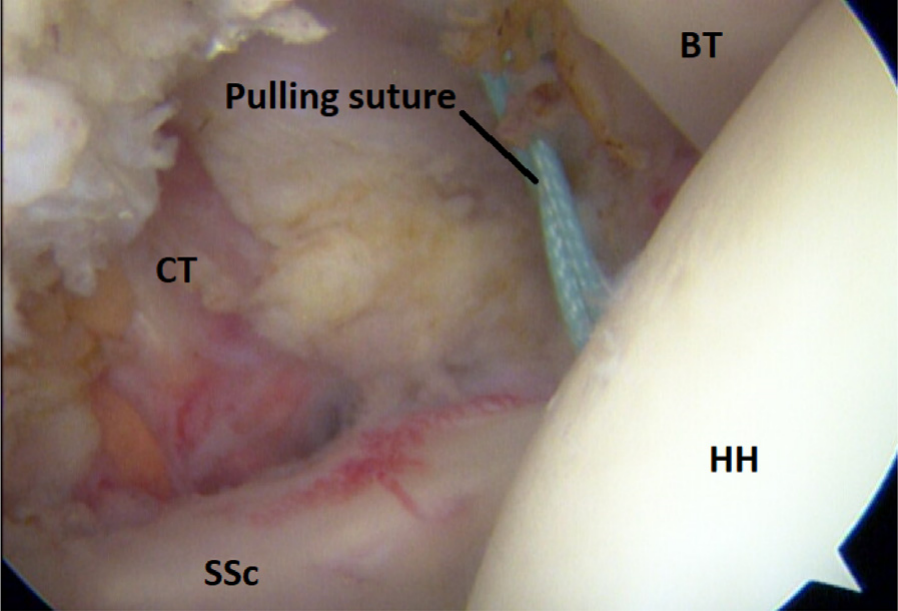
Arthroscopic view of a right shoulder, posterior viewing portal. Ti-cron #2 pulling suture situated posterior and anterior of the subscapular tendon and lateral of the conjoined tendon (CT). (BT, biceps tendon; HH, humeral head; SSc, subscapular tendon).
